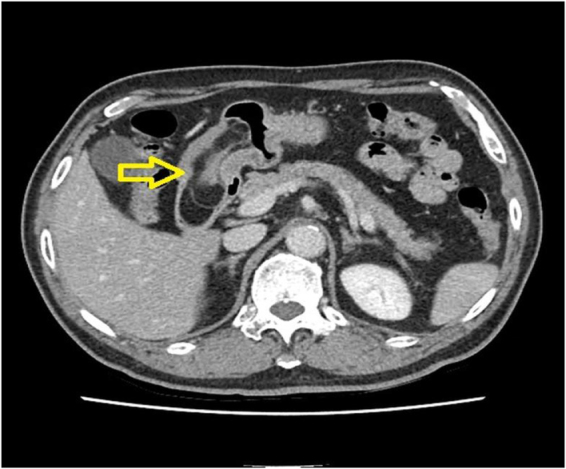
Pre-operative CT, multiseptated fat-density mass (8.1 × 2.7 × 3.8 cm) centered on the distal antrum/pyloric channel, traversing into the duodenal bulb with segmental luminal narrowing.
